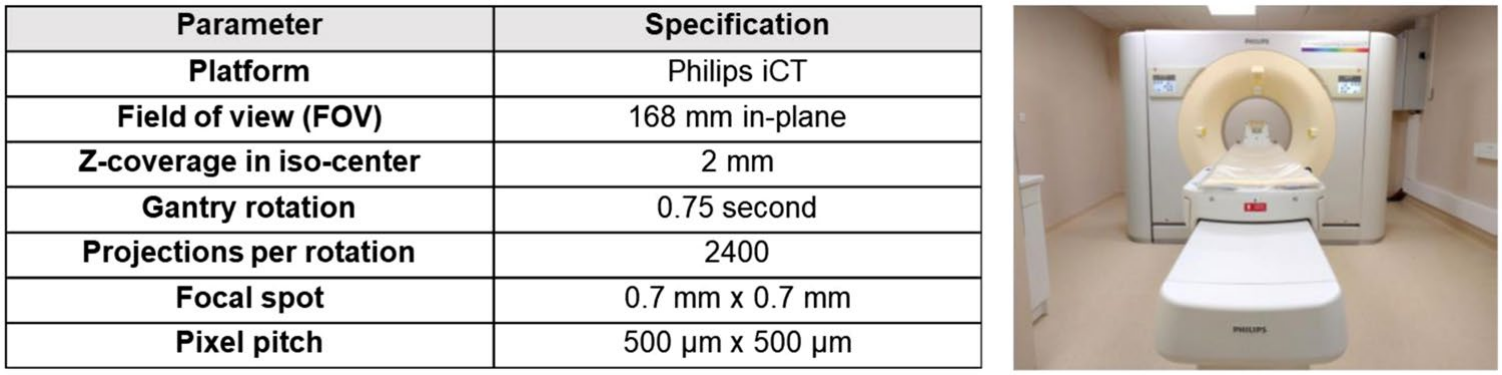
Specifications and photograph of the prototype photon-counting CT scanner used in this study.
